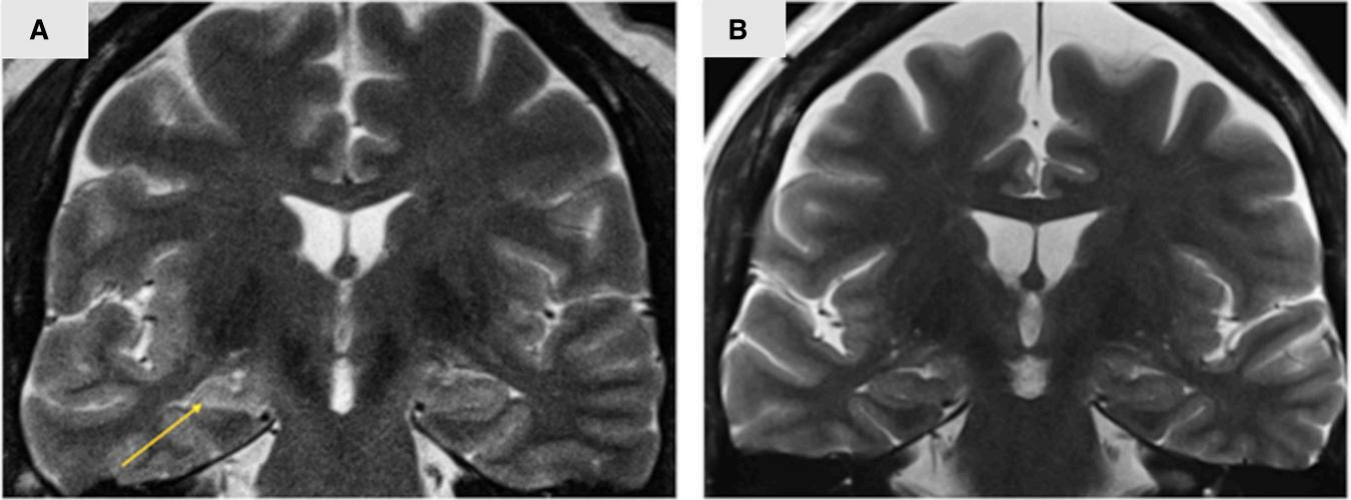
MRIs of a monozygotic twin pair concordant for MTLE and antecedent febrile seizure but discordant for hippocampal sclerosis. Right hippocampal sclerosis (HS) was demonstrated only in twin (A) with a history of prolonged febrile seizures. (B) Similarly positioned image from co-twin with no evidence of HS but with generalized parenchymal loss of undetermined cause. The MRI in Fig. 4A was taken at age 41 years before her epilepsy surgery, while her co-twin's MRI in Fig. 4B was taken at age 54 years. This twin pair was not included in our previous study of monozygotic twins with HS.^11^ MTLE = mesial temporal lobe epilepsy.
